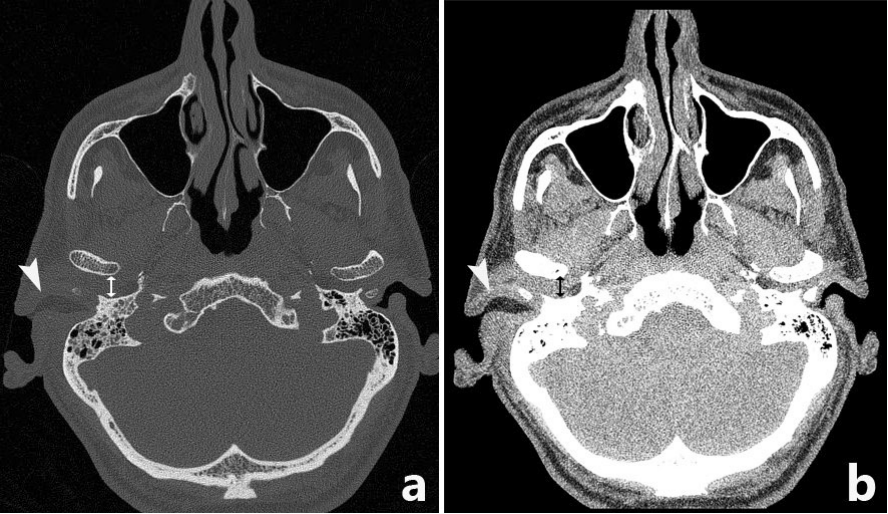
HRCT widening of temporomandibular joint. A 67-year-old male presented after 5 weeks with signs of external otitis and pain during chewing, without improvement after admission of topical antibiotics. An EAC polyp was removed. Bacterial culture revealed *Pseudomonas aeruginosa*. A non-contrast HRCT of the temporal bone was performed. The figure shows a bone window (image a) and soft tissue window (image b). Non-contrast-enhanced CT shows thickening of soft tissue of the EAC (image b arrow) as well as an anteriorly displacement of the mandible head with widening of the temporomandibular joint (black double arrow), indicating anterior spread of inflammation to the TMJ. At this point, no signs of bone erosion were present. Symptoms resolved after 4 weeks of iv. antibiotic therapy
